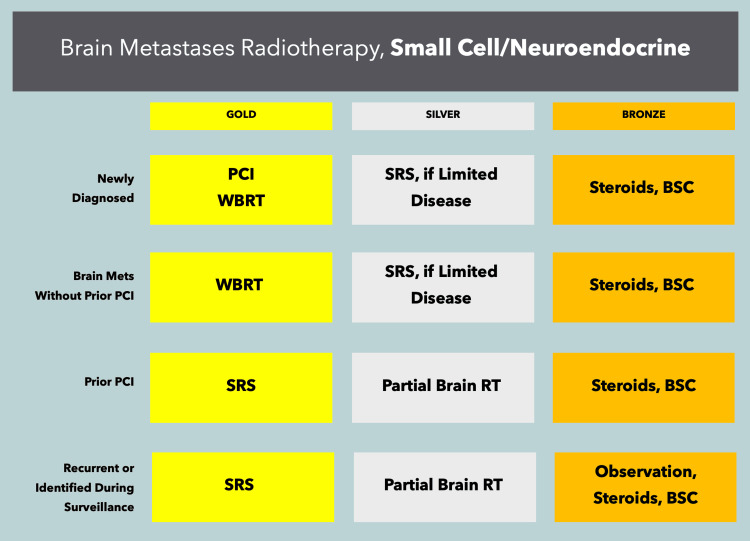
ACROPath® recommendations for the treatment of brain metastases from small cell and neuroendocrine malignancies PCI: prophylactic cranial irradiation, WBRT, whole-brain radiotherapy, SRS, stereotactic radiosurgery or fractionated stereotactic radiosurgery, RT: radiation therapy, BSC: best supportive care. For clarity, PCI regimens should not be used for patients who have radiographic evidence of existing metastatic brain disease, but rather as a preventative strategy in patients who are at risk of developing brain metastases.
